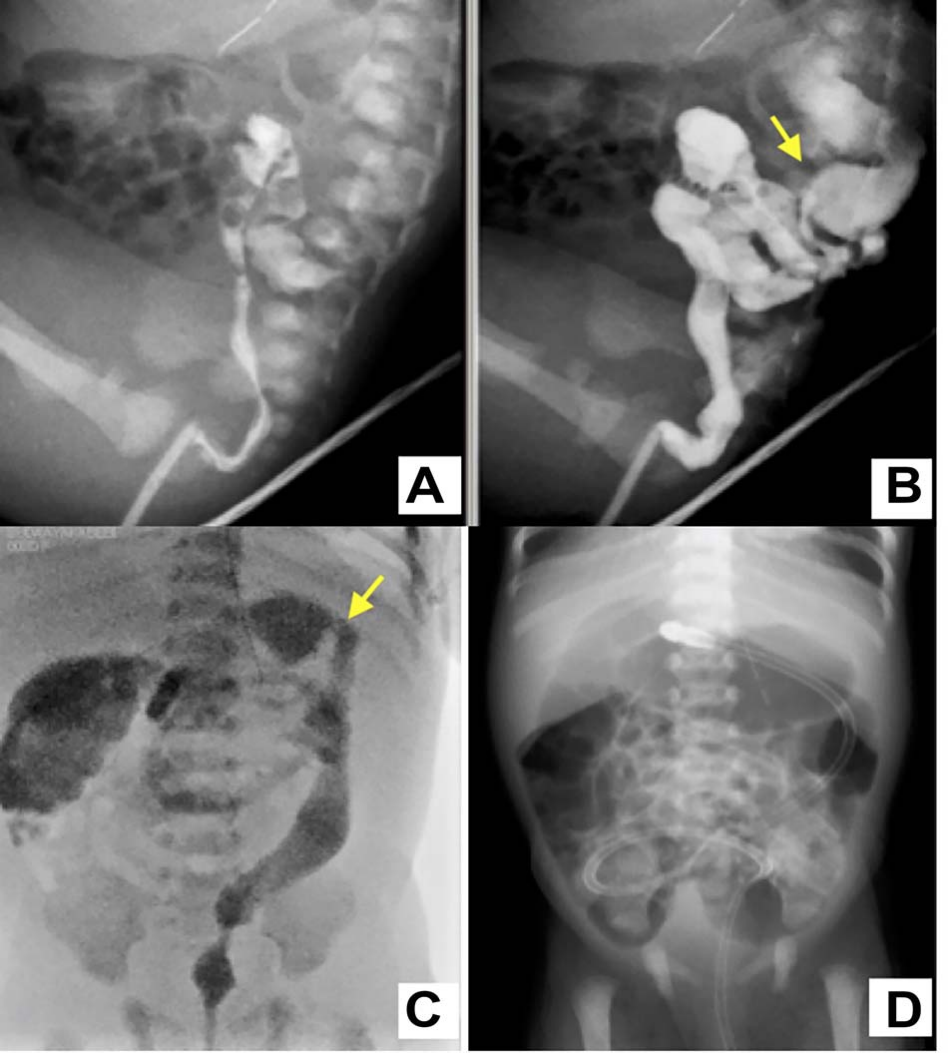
A water-soluble contrast enema image shows characteristic narrowing of the left colon up to the splenic flexure (yellow arrow) (A–C); a transanal tube was inserted into the transverse colon (C); the plain abdominal X-ray of the occluded tube shows dilation of the colon from the transverse colon to the oral side (D).
